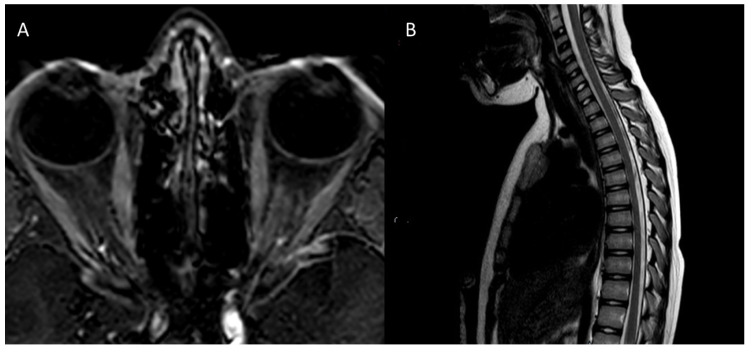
Magnetic resonance imaging scans of case 4. (A) Axial T1 post-gadolinium water selective orbital scan demonstrates a mild enhancement of the left optic nerve lasting 45 days after the onset. (B) Sagittal spinal cord T2 weighted scan shows the absence of medullary demyelinating lesions, with transverse myelitis being more common as a recurrence than an onset symptom of neuromyelitis optica.
